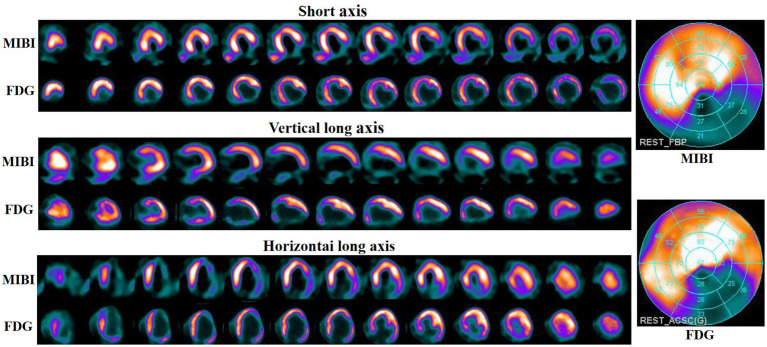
Rest myocardial perfusion ^99m^Tc-MIBI SPECT images demonstrating match with ^18^F-FDG PET/CT images in patient with CHD. SPECT shows severe perfusion defect in inferior, inferolateral walls and apical, and PET shows predominant absence of ^18^F-FDG uptake, thus, findings consistent with scarred myocardium.
